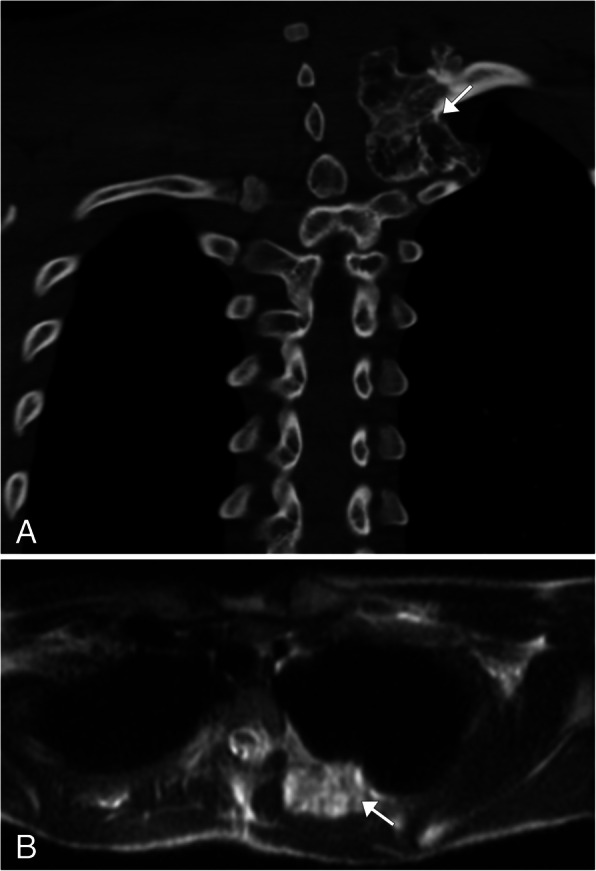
Osteochondroma. a Coronal CT image of the chest showing an exostosis involving the medial aspect of the posterior first rib (arrow). b Axial T2WI MR imaging demonstrating a hyperintense continuous hyaline cap (arrow). There was no evidence of adjacent soft tissue involvement
